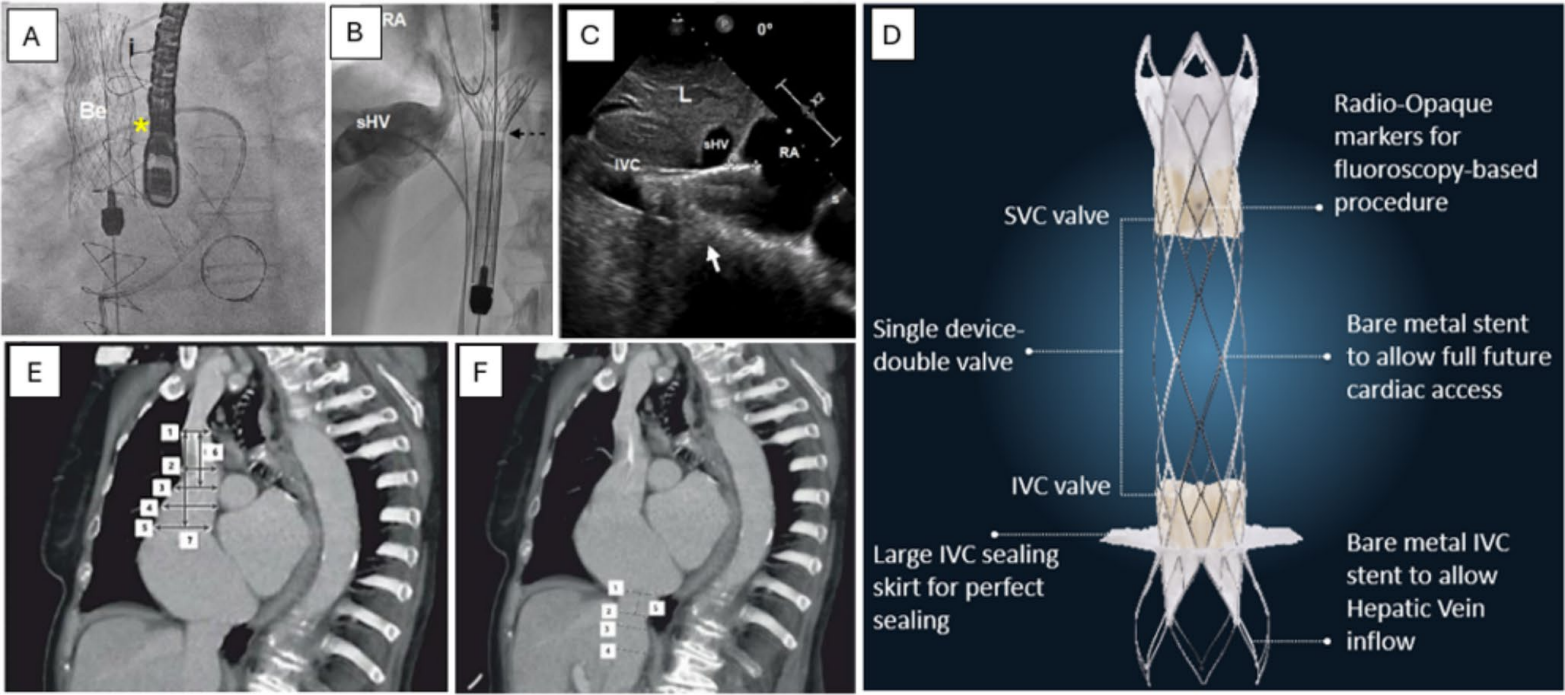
Legend. (A) The “belly” of the SVC stent graft should be implanted at the level of the right pulmonary artery, marked here with a Swan Ganz catheter (*star)*. (B) The IVC valve has a sealing skirt covering the proximal 20 mm of the stent; the radiolucent band marks where the skirt ends. (C) Optimal positioning should have 5 to 12 mm of the proximal edge of the IVC stent entering the RA. TEE (at 0$$\:^\circ\:$$ plane) shown here. *White arrow* indicates the inferior cavo-atrial junction. (D) Unica (Innovalve), a single-device, dual-anchoring caval stent with two bioprosthetic valves. Open struts at the right atrial face permit easier cardiac access for subsequent procedures. (Fig. 1D with permission from Innoventric.) (E, F): SVC and IVC measurements in CT sizing for TricValve. Relevant SVC diameter measurements (from top to bottom) are at the confluence with the innominate vein; at the top, middle, and bottom levels of the pulmonary artery; and at the superior cavo-atrial junction. Orthogonal lengths from innominate confluence to mid pulmonary artery level and from innominate confluence to superior cavo-atrial junction are also recorded. Relevant IVC diameter measurements (from top to bottom) are at the inferior cavo-atrial junction; the top and bottom of the hepatic vein confluence, and at 5 cm inferior to the inferior cavo-atrial junction. Orthogonal length from inferior cavo-atrial junction to the top of the hepatic vein confluence is also recorded. (Figures A-C, E-F from: J Clin Med. 2021;10:4601; https://www.mdpi.com/2077-0383/10/19/4601; Creative Commons user license https://creativecommons.org/licenses/by/4.0/) [20]. Be: belly of SVC stent graft; RA: right atrium; sHV: superior hepatic vein; IVC: inferior vena cava; SVC: superior vena cava
